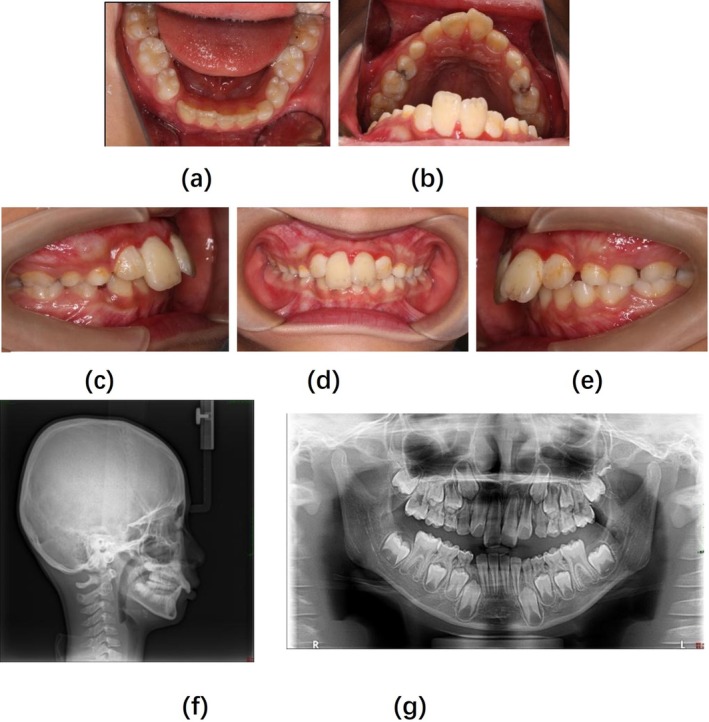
Clinical and radiograph view 12 months after surgery. (a–e) Intraoral view 12 months; (f–g) radiograph view 12 months.
